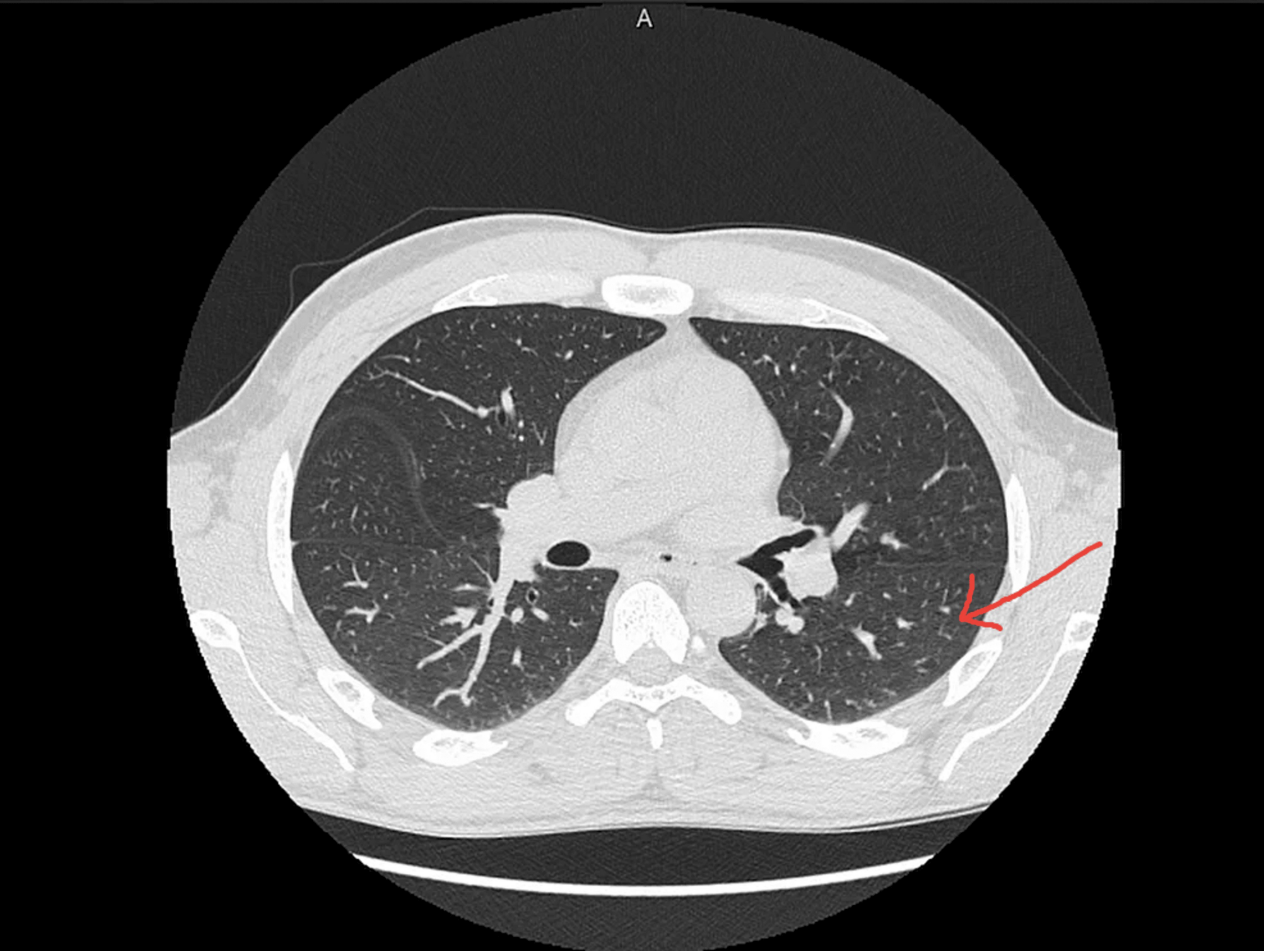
Follow-up CT scan of the chest Done on August 11, 2023.
